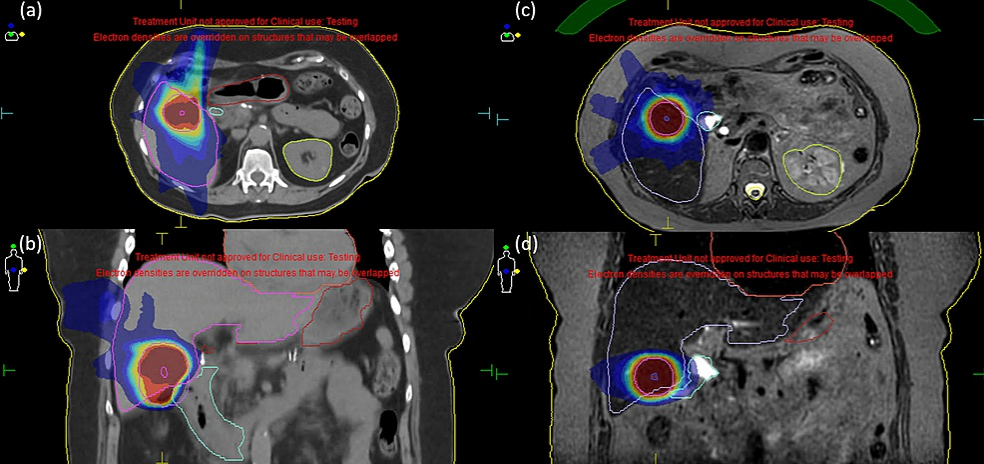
Isotoxic planning comparisons between CTgRT and MRgOART for case three. The tumor was very small, and a large CT-PTV expansion of 10 mm was required to obtain a BED10 <100 Gy.
